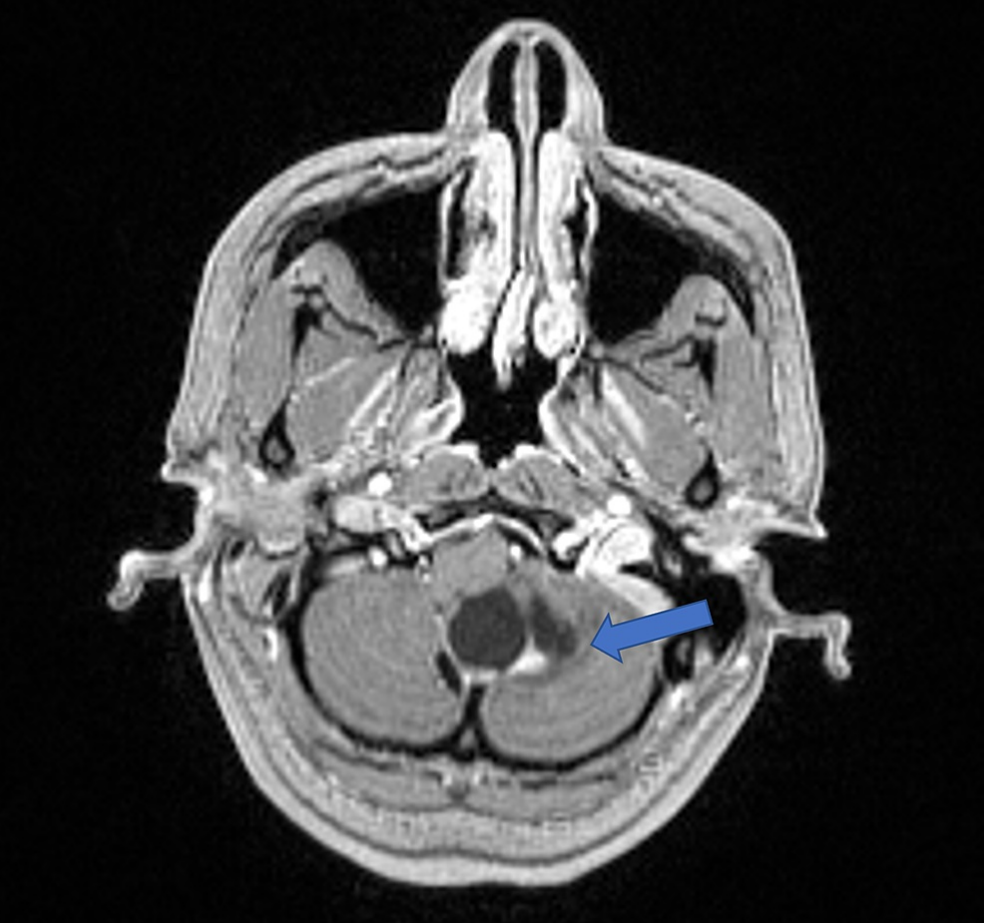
Axial T1 MRI with contrast Six mm solid enhancing component in the inferior aspect of the lesion (blue arrow)
